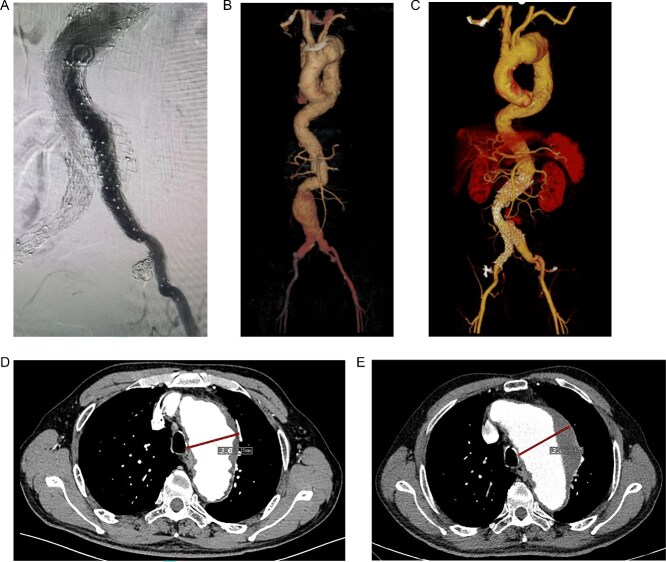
(A) Internal iliac artery embolization and stent graft implantation were performed. (B) Preoperative aortic CTA in 2019. (C) Type Ib endoleak from left iliac artery stent graft. (D) CTA shows the diameter of aortic arch aneurysm was 61.15 mm in July 2019. (E) CTA shows the diameter of aortic arch aneurysm was 72.64 mm in July 2022.
